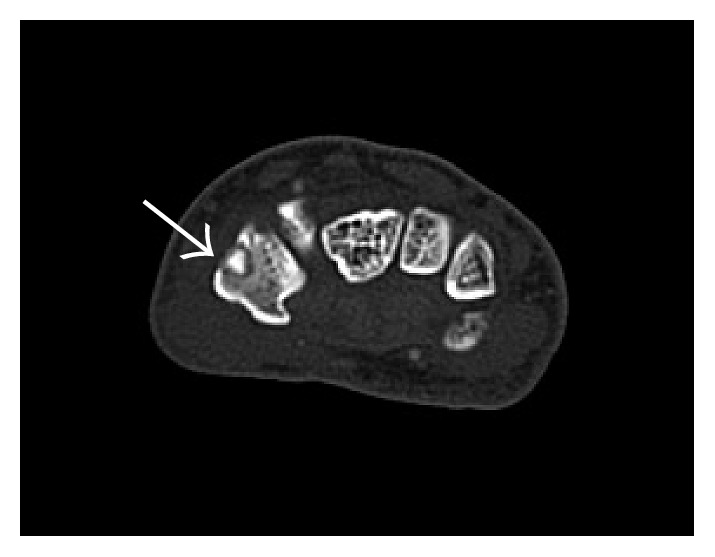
Computed tomography showing sclerotic nidus surrounded by radiolucent halo (arrow).
